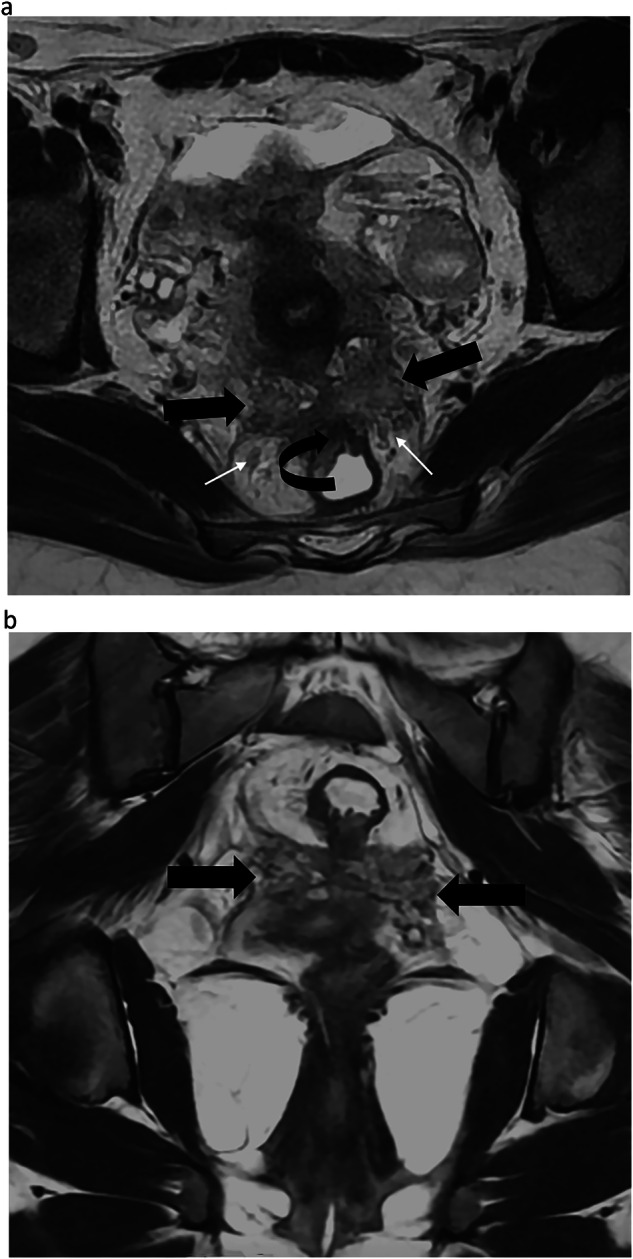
Endometriosis in a 39-year-old woman with deep dyspareunia, chronic constipation, and weak urinary flow. a Axial T2-weighted MR image shows a retractile fibrotic mass (straight arrows) involving the torus uterinus, uterosacral ligaments, pouch-of-Douglas (mediocentral compartment), and rectum (curved arrow) (posterocentral compartment), with bilateral mediolateral (dark arrow) and posterolateral parametrium (white arrow). Bilateral involvement of the inferior hypogastric plexus, hypogastric nerves (white arrows), and splanchnic roots is also noted. b Coronal T2-weighted MR image shows the parametrial extension of the lesions (arrows). Thus, this patient is quoted with a dPEI score of 6 (severe disease) with 6 compartments affected (mediocentral, posterocentral, right and left mediolateral, right and left posterolateral)
